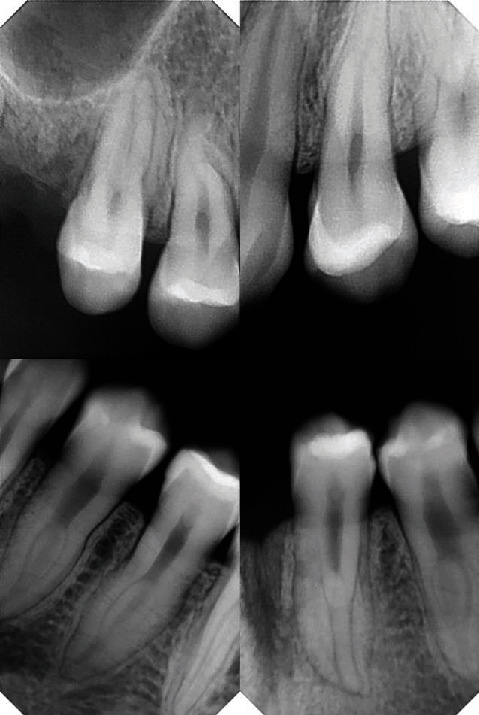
Digital periapical radiographs of the patient's premolars.
